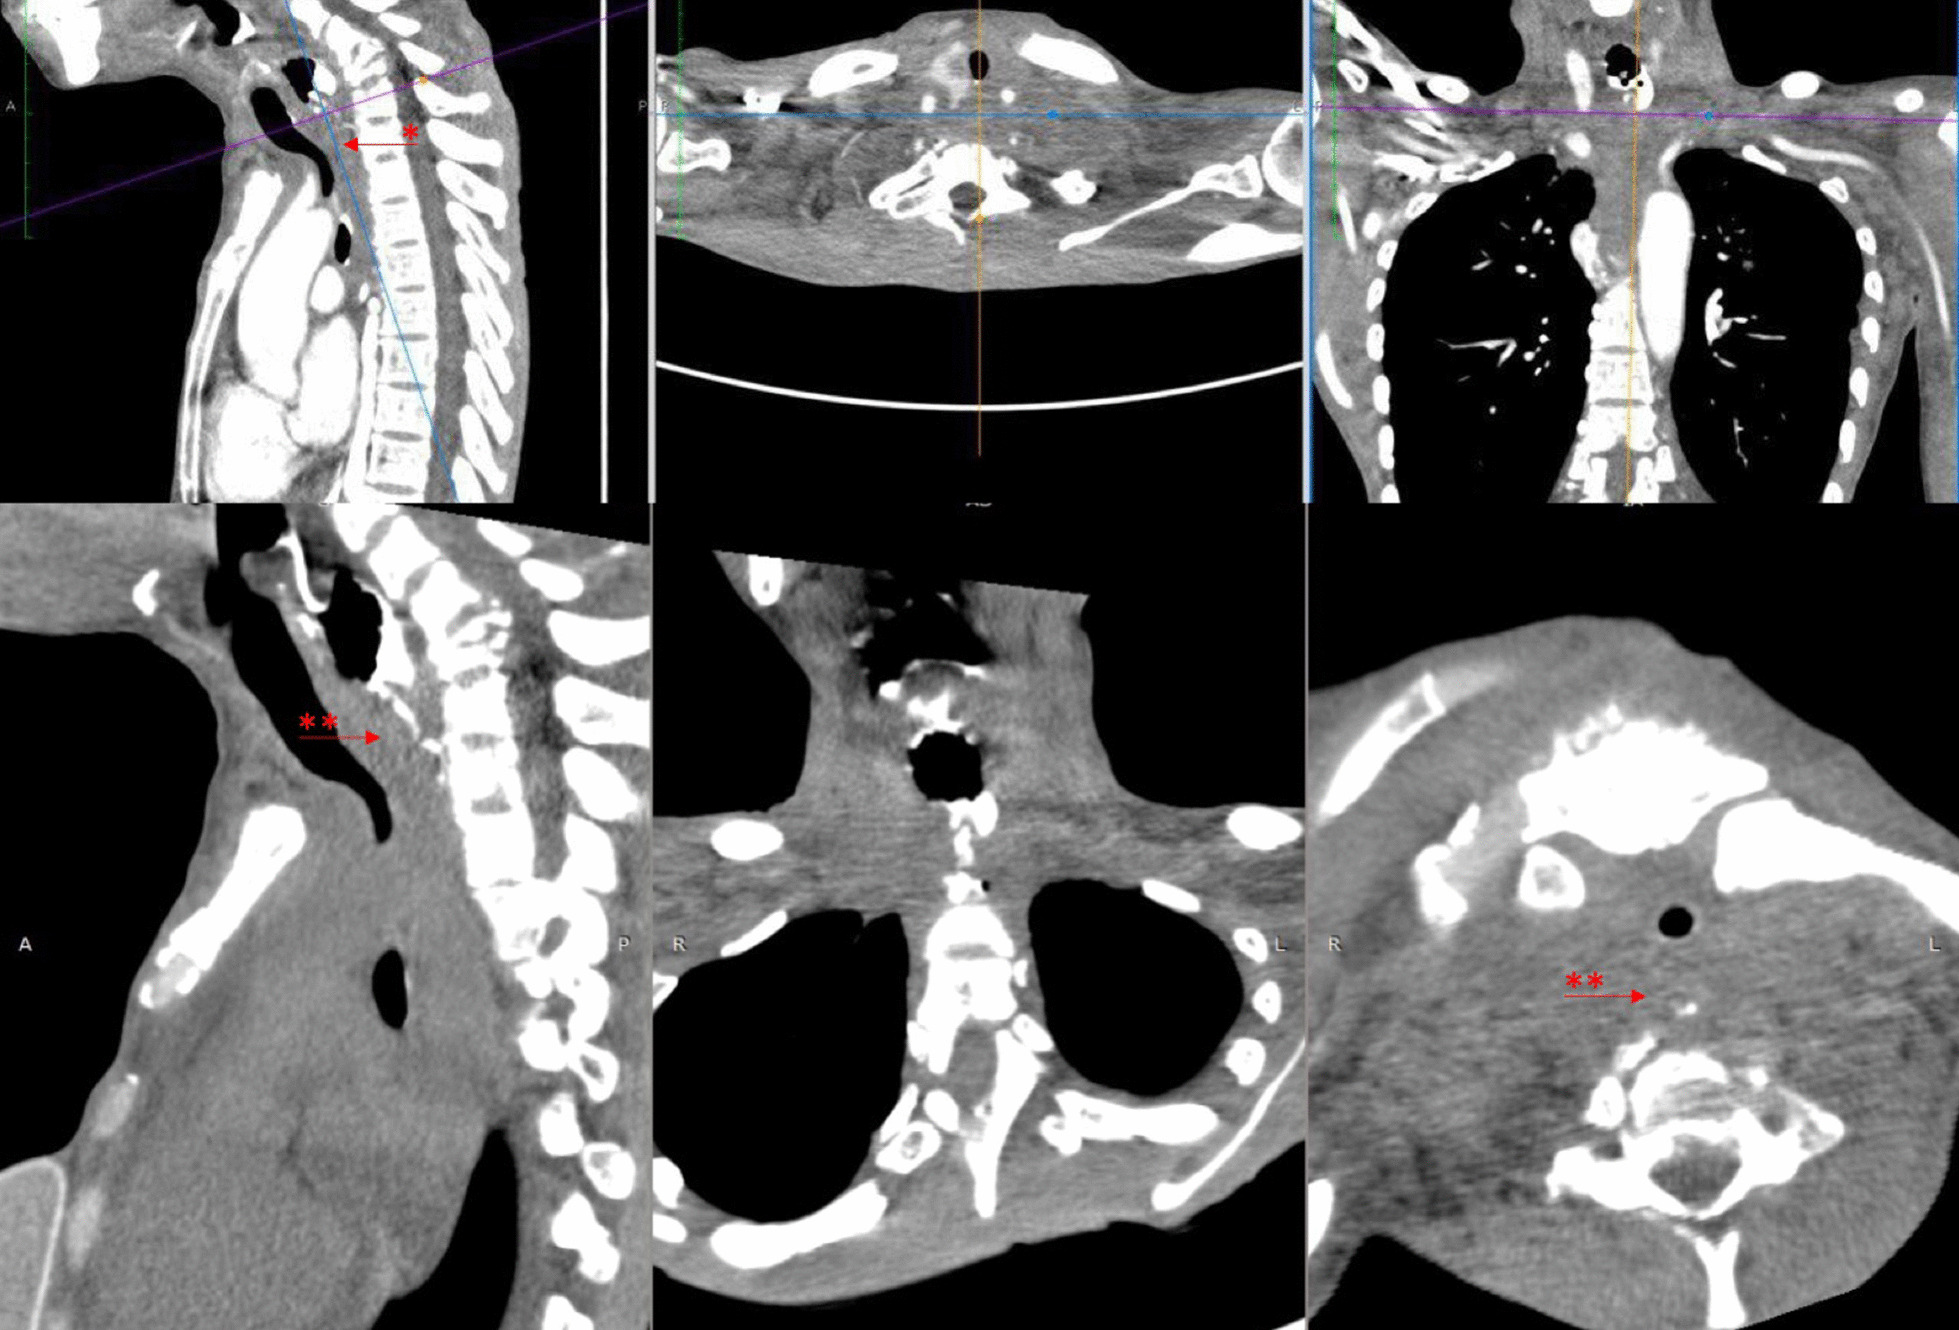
Neck computed tomography scan with oral contrast shows filling defect in the cervical part of the esophagus (in the projection of fifth cervical to first thoracic vertebrae) (*). The amorphous lesion with irregular border appears to compress the esophageal lumen ()
